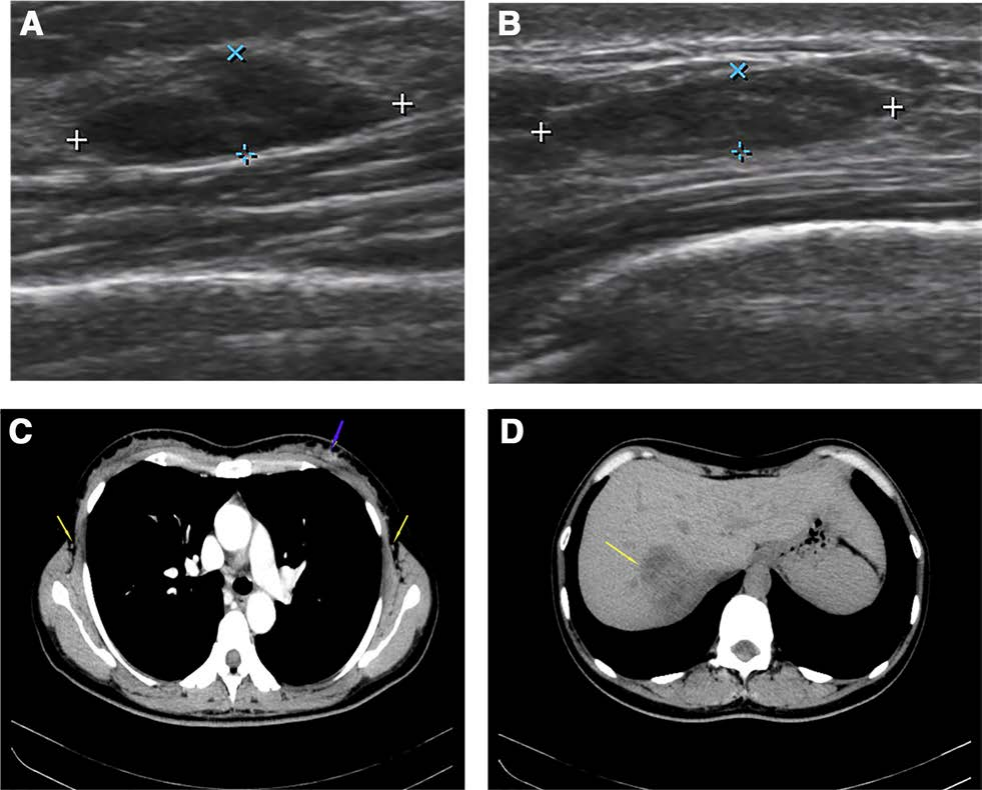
Case 1: Images of breast nodules and liver masses. The tissue structure of each layer of the bilateral breast was clear, the glandular tissue was relatively homogeneous, with a strong echo of fine honeycomb, and hypoechoic nodules were seen in the glands. The maximum left side was about 13 mm × 4 mm (12 o’clock direction, about 20 mm from the nipple). (A) The maximum right side was about 14 mm × 4 mm (7 o’clock direction, beside the areola). (B) Oval, parallel growth, complete edge, uniform internal echo, no punctate strong echo, the superficial fascia of the breast at the lesion was shallow and deep, and there was no obvious blood flow signal in the nodules. (C) Enhanced CT showed uneven density of the left breast, multiple punctate enhanced nodules in the left breast area with clear edges (purple arrows), and multiple small lymph nodes in the bilateral axilla (yellow arrows). (D) Plain CT scan showed multiple nodular, lumpy slightly low-density lesions (yellow arrows) in the right lobe of the liver, partially fused into sheets, with unclear boundaries, and a range of about 50 mm × 42 mm.
